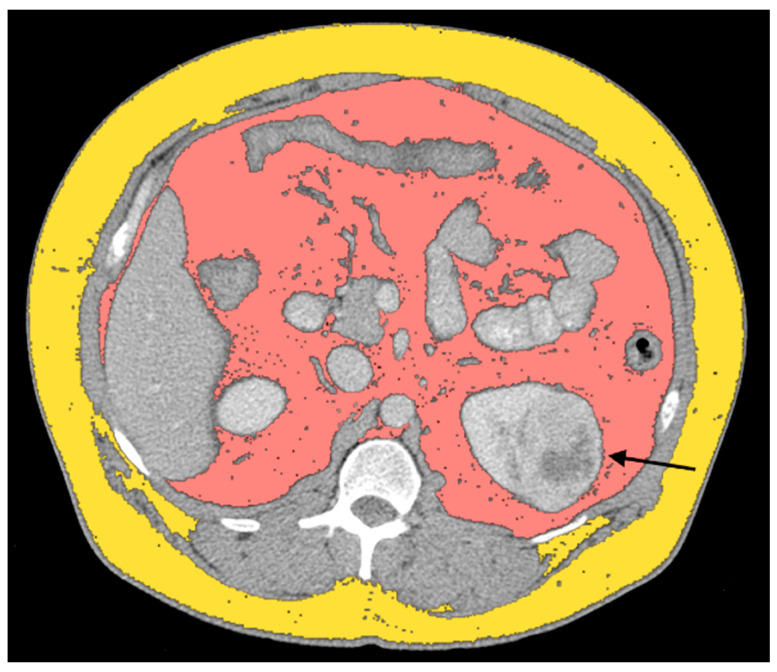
Axial CT image showing ccRCC with *VHL* mutation (black arrow), SAT, and VAT, segmented, respectively, in dark yellow and salmon pink.
